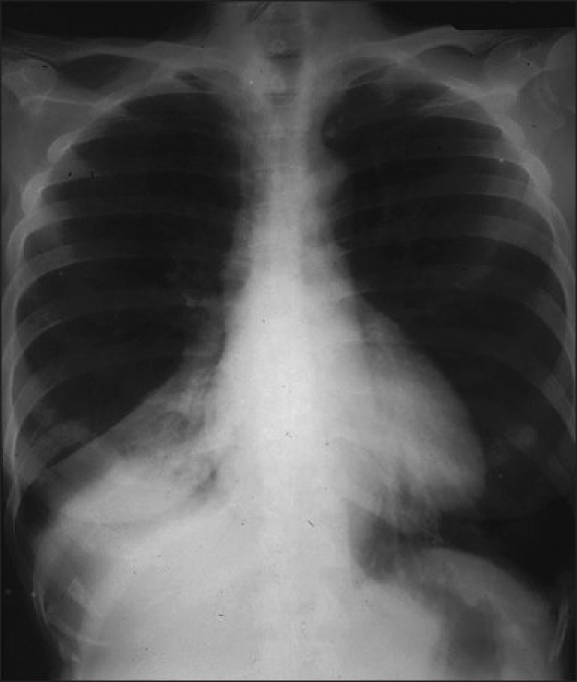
An AP chest radiograph showing atelectasis of the right lower lobe. Note that the collapsing lobe has moved centrally and inferiorly towards the lower dorsal spine, where it is seen as a triangular opacity partially silhouetting the right hemidiaphragm and associated with a subtle air bronchogram. The minor fissure shows inferior displacement. Right–lower-lobe atelectasis can be differentiated from right–middle-lobe atelectasis by the persistence of the right heart border as in this case
